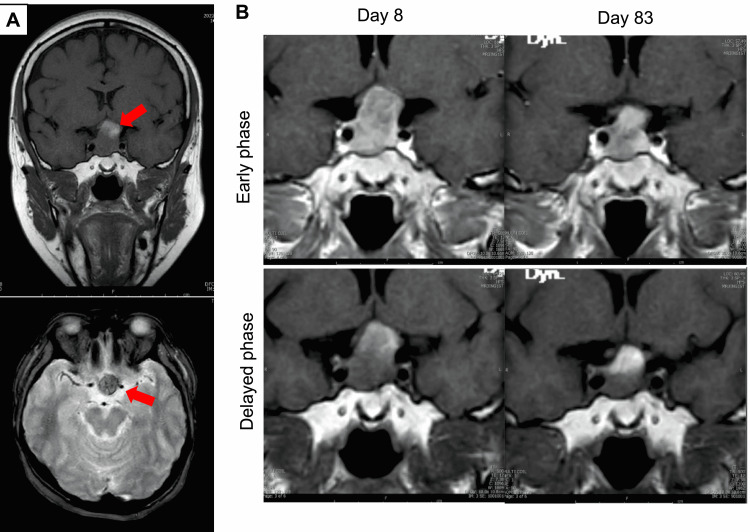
Magnetic resonance imaging (MRI) of the case. (A) Magnetic resonance (MR) image of the pituitary adenoma on the day of onset (Upper panel: coronal, T1W, Lower panel: horizontal, T2W. Red arrows indicate the pituitary adenoma). (B) Dynamic contrast-enhanced image of macroprolactinoma on day 8 (left panel) and day 83 (right panel). Early phase (upper panel) and delayed phase (lower panel) are shown.
